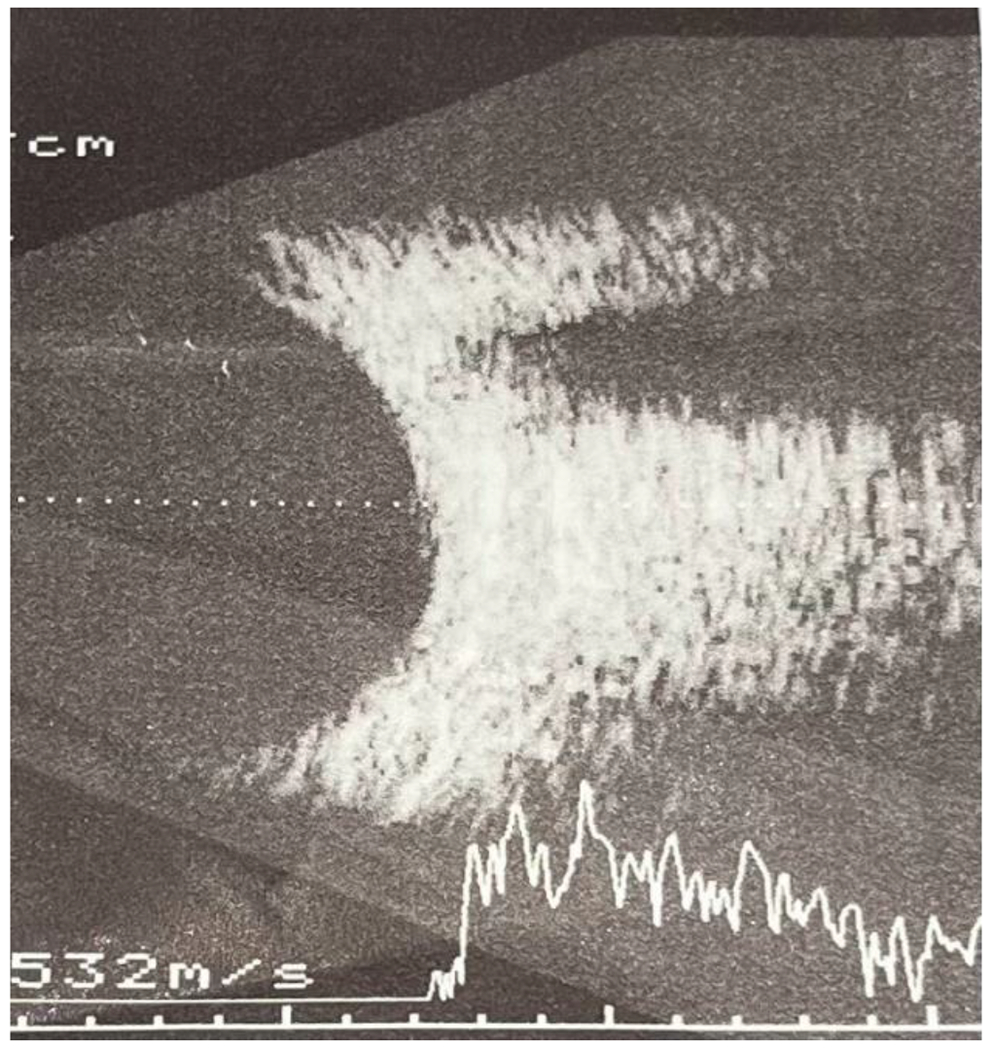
Ocular ultrasonography of the RE within the normal range. Source: Own creation.
